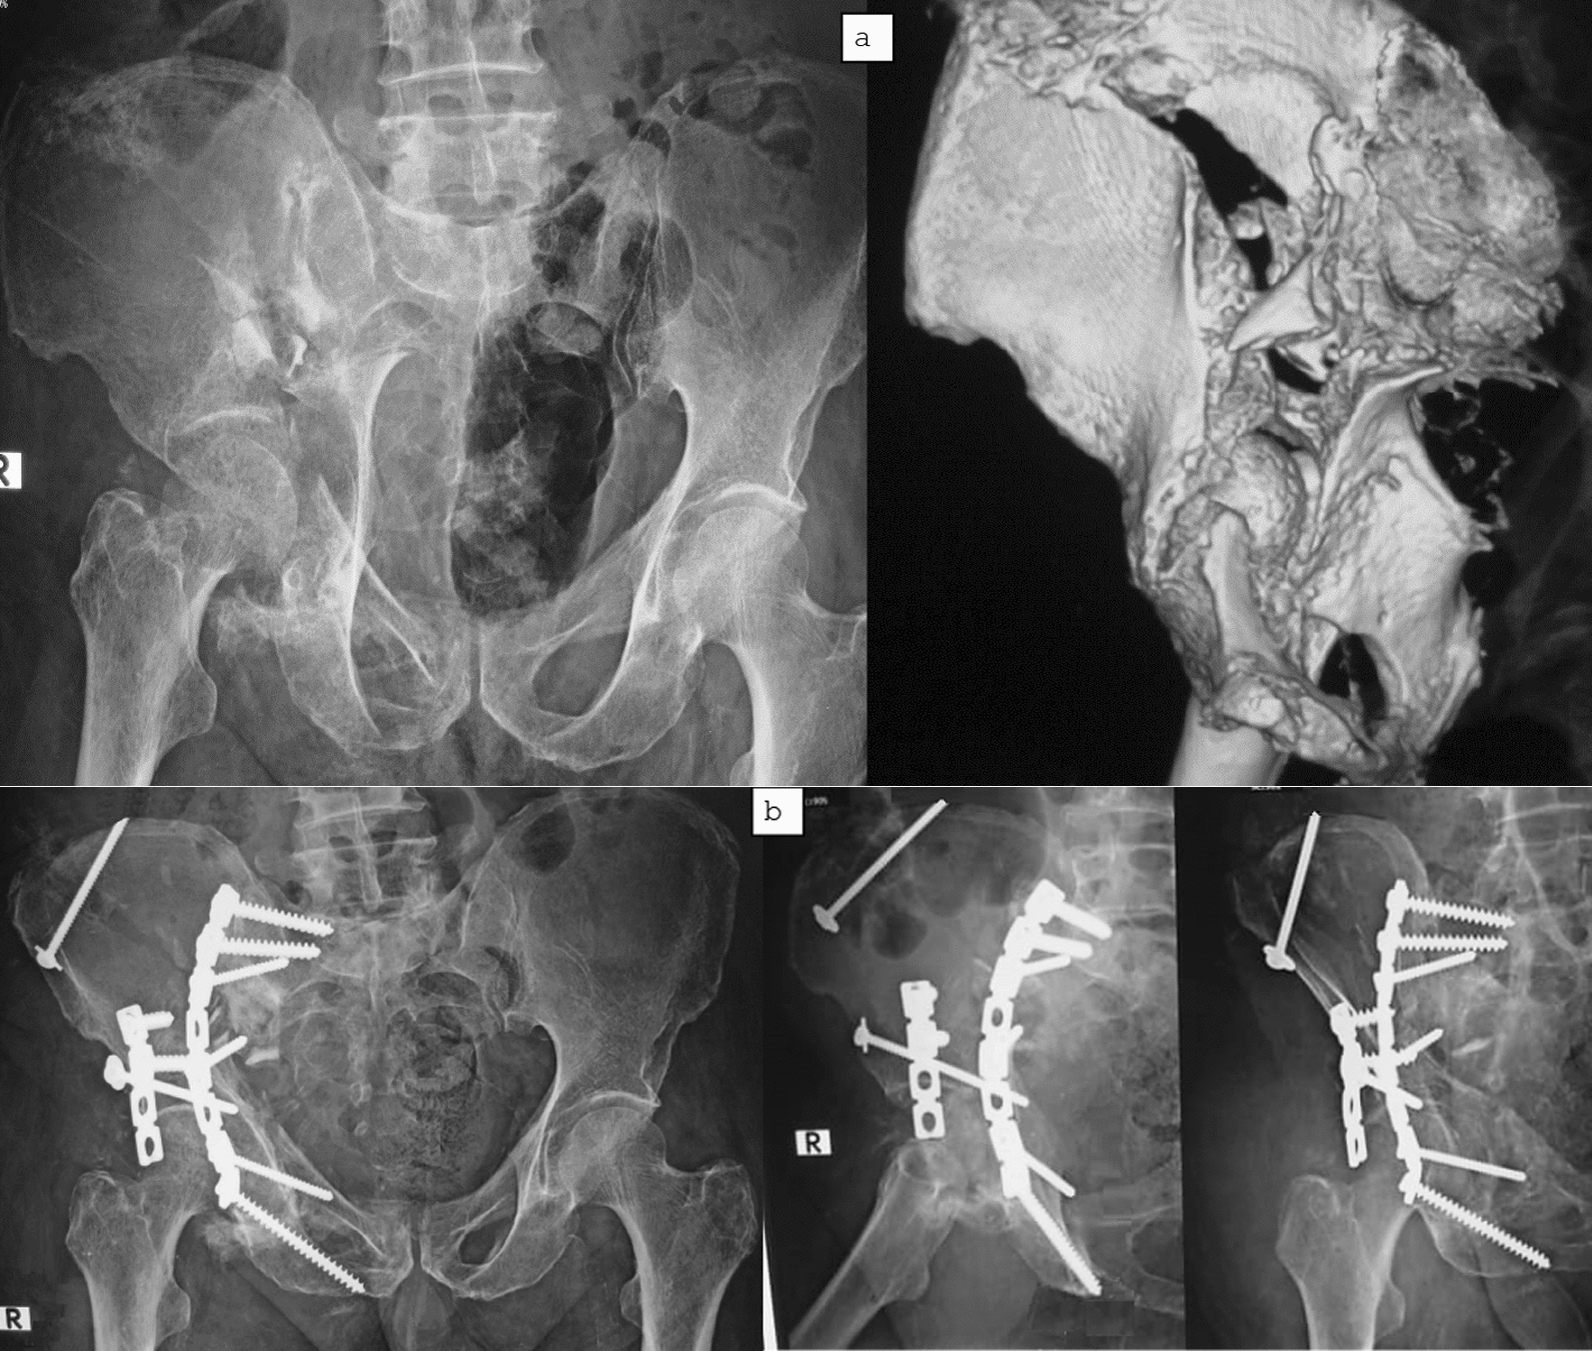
A 36-year-old male with a 5-week-old neglected both-column acetabular fracture. a Preoperative radiograph 3D CT showing displaced comminuted fracture with early callus. b Postoperative radiograph at the final follow-up visit showing acceptable reduction and fixation, with restoration of hip congruity
